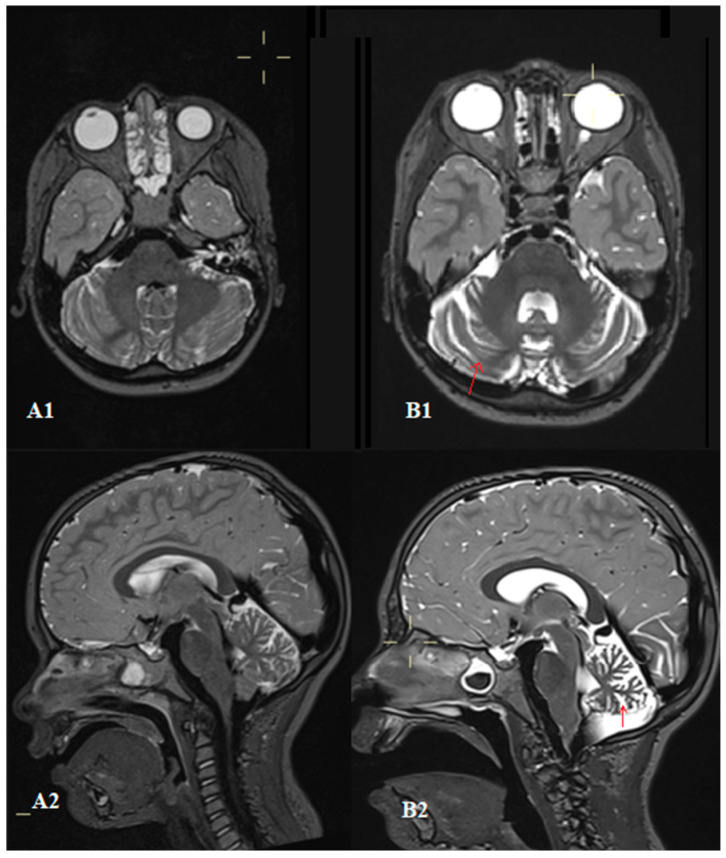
T2-weighted MRI sequences of the brain demonstrate bilateral cerebellar hemispheric and vermis atrophy (B1,B2) in comparison to prior imaging performed three years before, when these structures appeared normal (A1,A2). The red arrow represents cerebellar athrophy.
